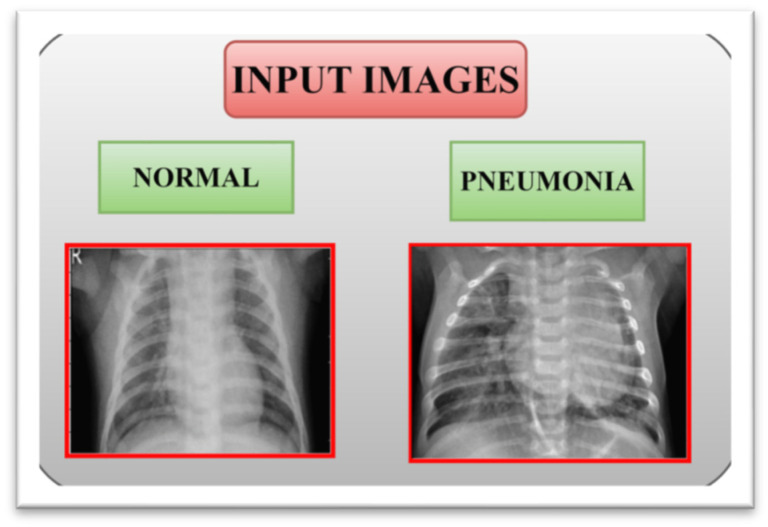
Sample chest X-ray image of normal and pneumonia.
